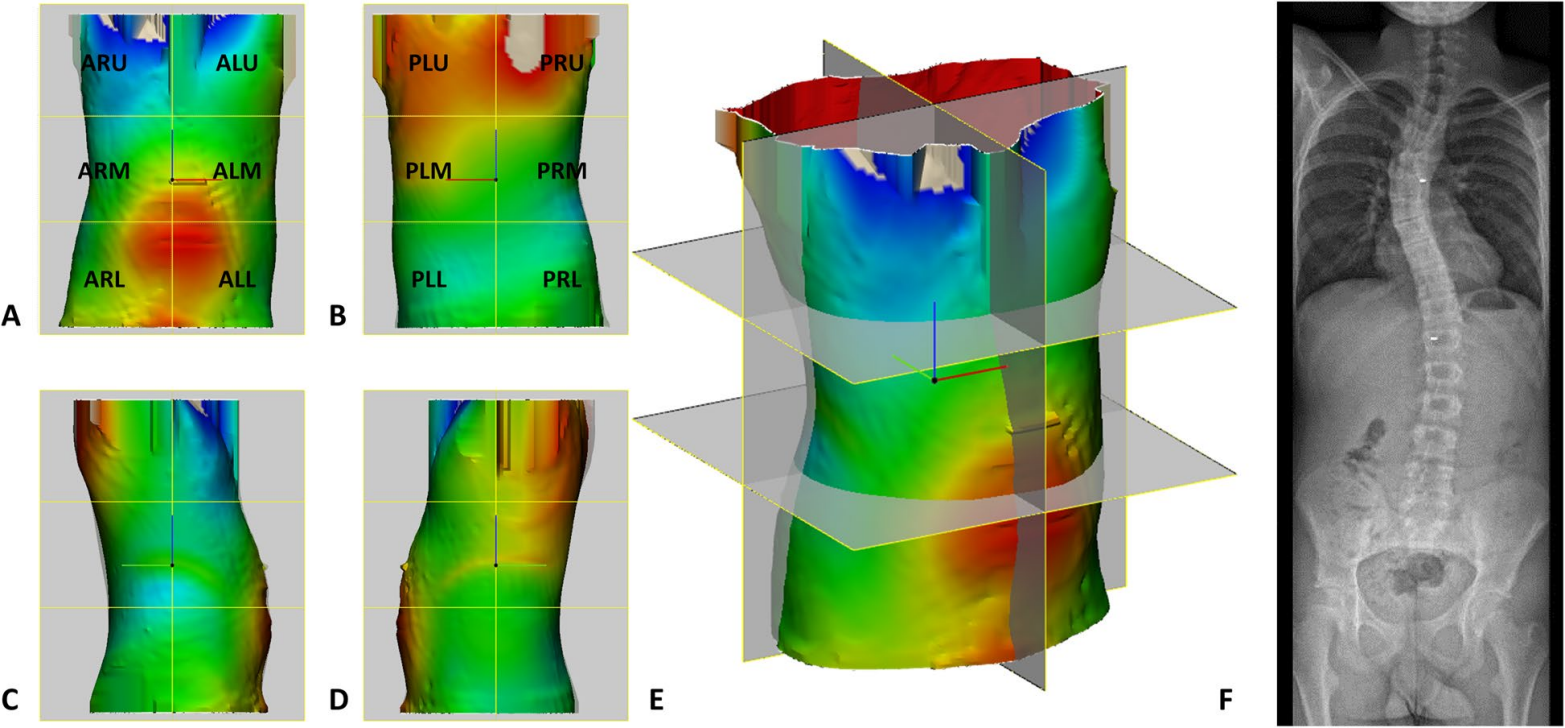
Surface-to-surface measurements (A-E) and EOS radiograph (F) of a typical case, in A) anterior, B) posterior, C) right, D) left, E) perspective view. Initial in-brace correction = 9.6%. Abbreviations: ALU anterior left upper segment ARU anterior right upper segment, ALM, anterior left midsegment, ARM, anterior right midsegment, ALL, anterior left lower segment, ARL, anterior right lower segment, PLU, posterior left upper segment, PRU, posterior right upper segment, PLM, posterior left midsegment, PRM, posterior right midsegment, PLL, posterior left lower segment, PRL, posterior right lower segment.
